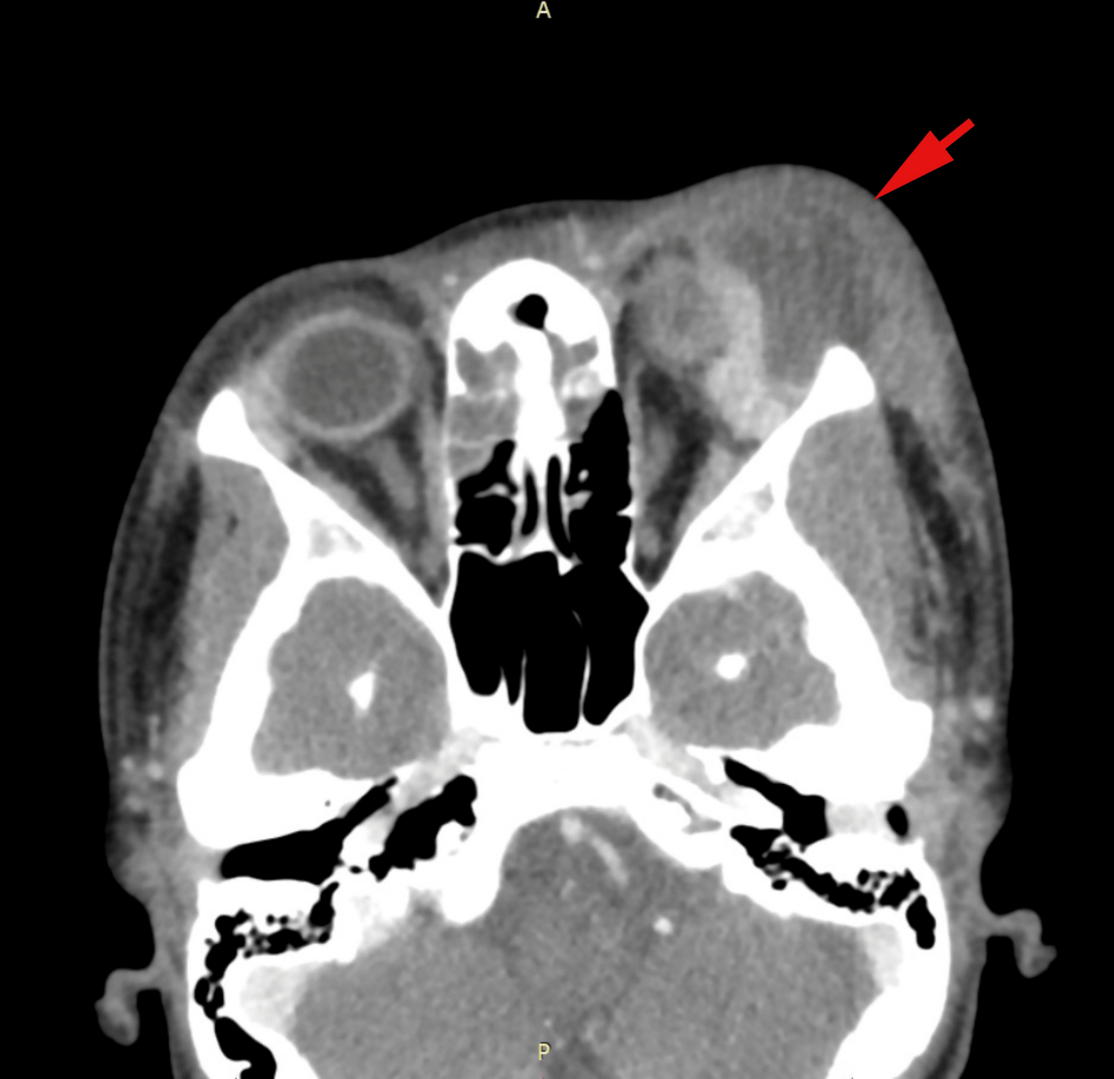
CT of left orbital and frontal subperiosteal abscesses Axial contrast-enhanced CT scan demonstrating a 3.1 x 2.6 x 2.5 cm subperiosteal abscess in the left orbit, causing mass effect on the globe, superior rectus, and lacrimal gland. A secondary abscess (1.7 x 0.7 x 1.9 cm) is noted along the left frontal bone. Marked periorbital edema and near-total opacification of the frontal and ethmoid sinuses are evident.
